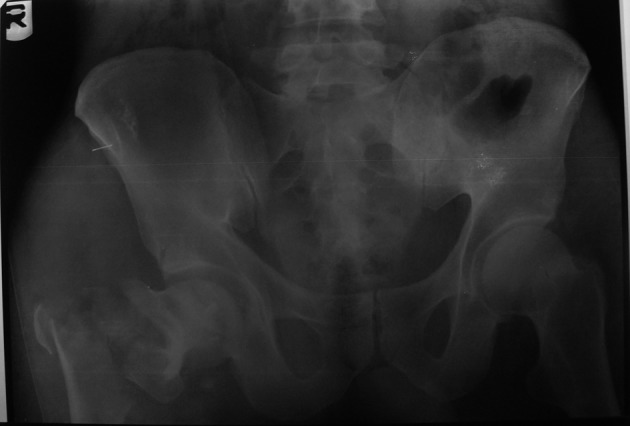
Radiograph Taken on Admission
